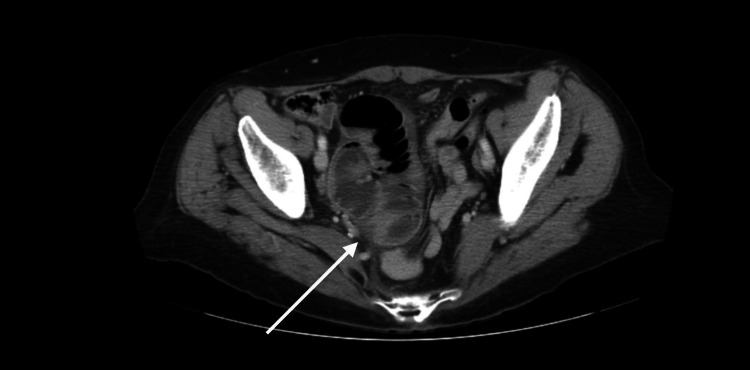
CT of the abdomen shows prominent mid-ileal loops with torsion of ileal loops around its mesentery, suggestive of probable mid-ileal volvulus (white arrow). CT: computed tomography
